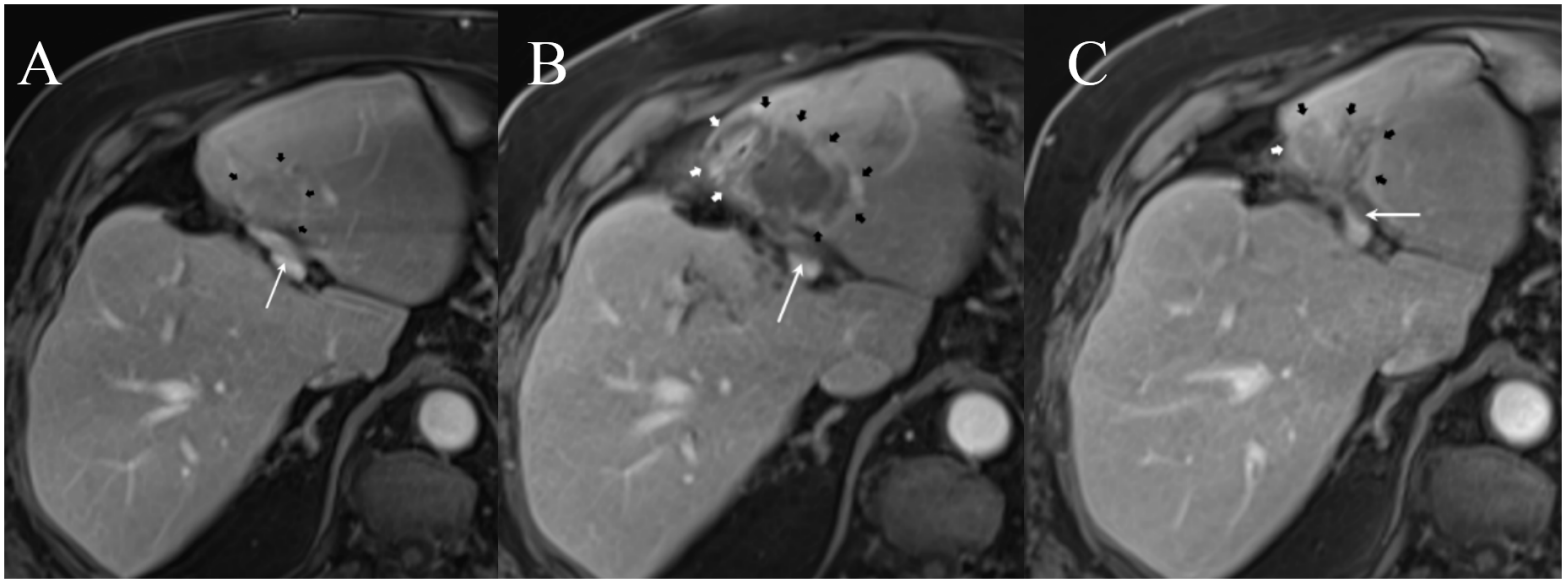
A 75-year old man (patient #15) with hepatocellular carcinoma. (A) Pre-interventional contrast-enhanced T1w magnetic resonance imaging shows a freely perfused portal vein (thin arrow); HCC lesion is partly visible (thick arrows). (B) 1 day after the ablation, the T1w image shows the ablation zone (thick arrows) and newly occurred partial thrombosis of the left portal vein (thin arrow). (C) At the 4-month follow-up, partial thrombosis of the left portal vein has persisted (thin arrow), and the ablation zone has decreased (thick arrows).
